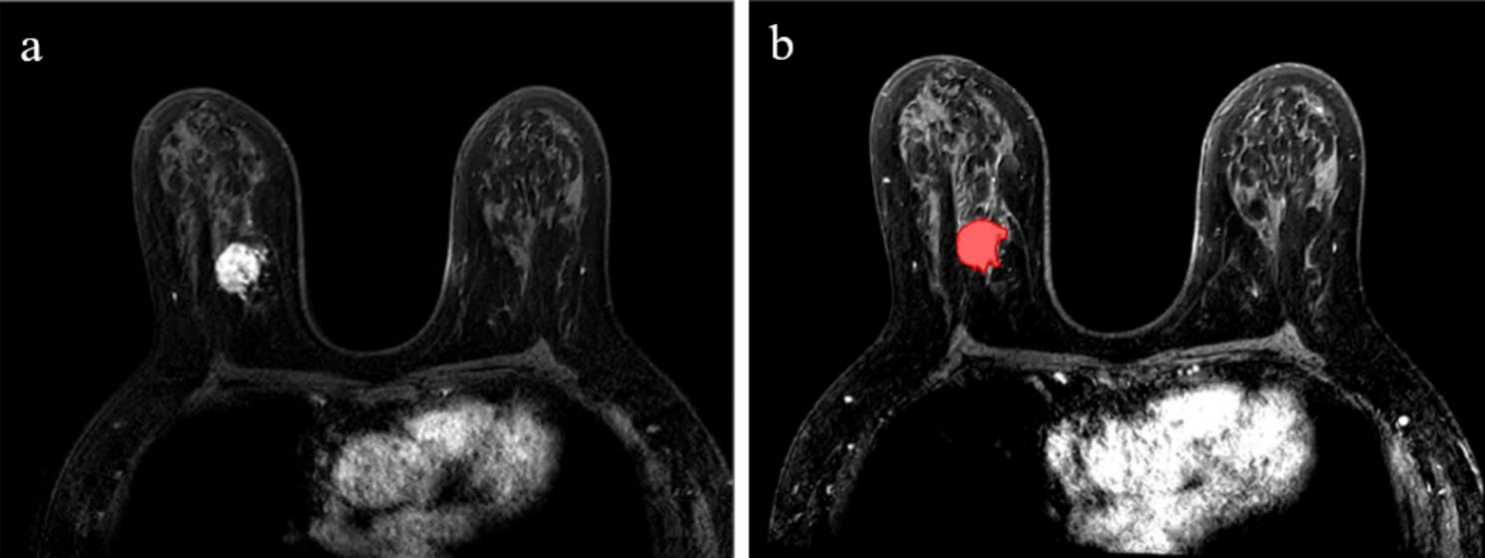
MRI images of a 56-year-old woman with breast cancer. (a) DCE postcontrast phase 2 image. (b) VOI of the primary tumor manually delineated by the radiologist
